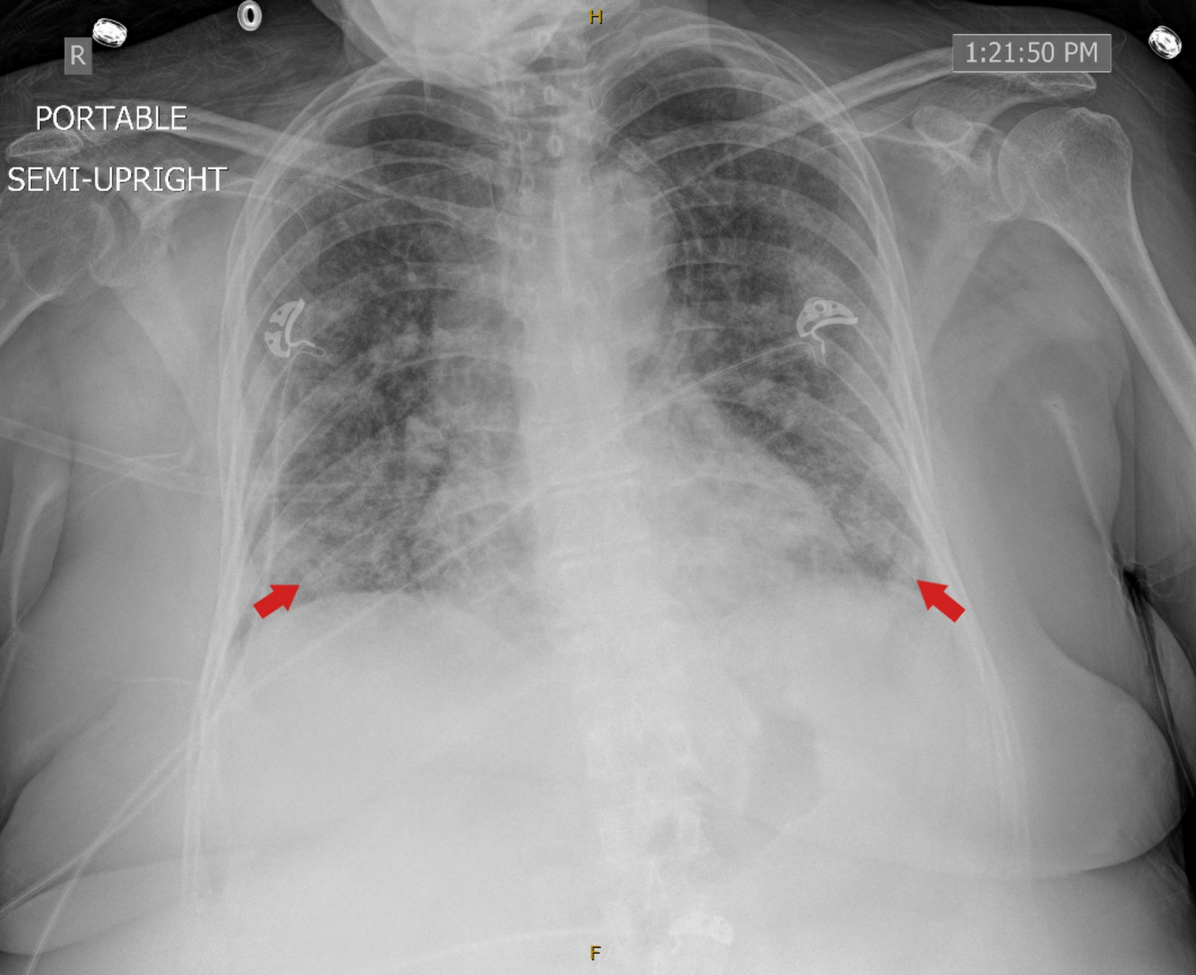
CXR on admission showed diffuse bilateral infiltrates. CXR, chest X-ray
